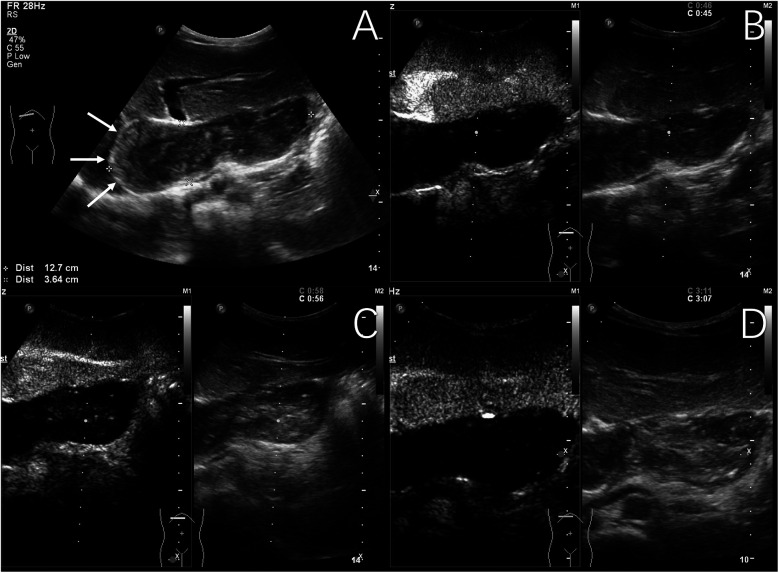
Typical ultrasound findings of hepatic cystic echinococcosis (case 1). Ultrasound of the liver revealed several mixed echogenic masses, with the larger one located in the caudate lobe of the liver, protruding between the left liver and stomach, with a maximum diameter of 127 mm, clear margin, irregular morphology, and patchy strong echoes detected around it (The white arrows show eggshell-like calcification around the lesion) (A) contrast-enhanced ultrasound examination showed no significant enhancement during the arterial phase (B), portal venous phase (C), and late phase (D).
